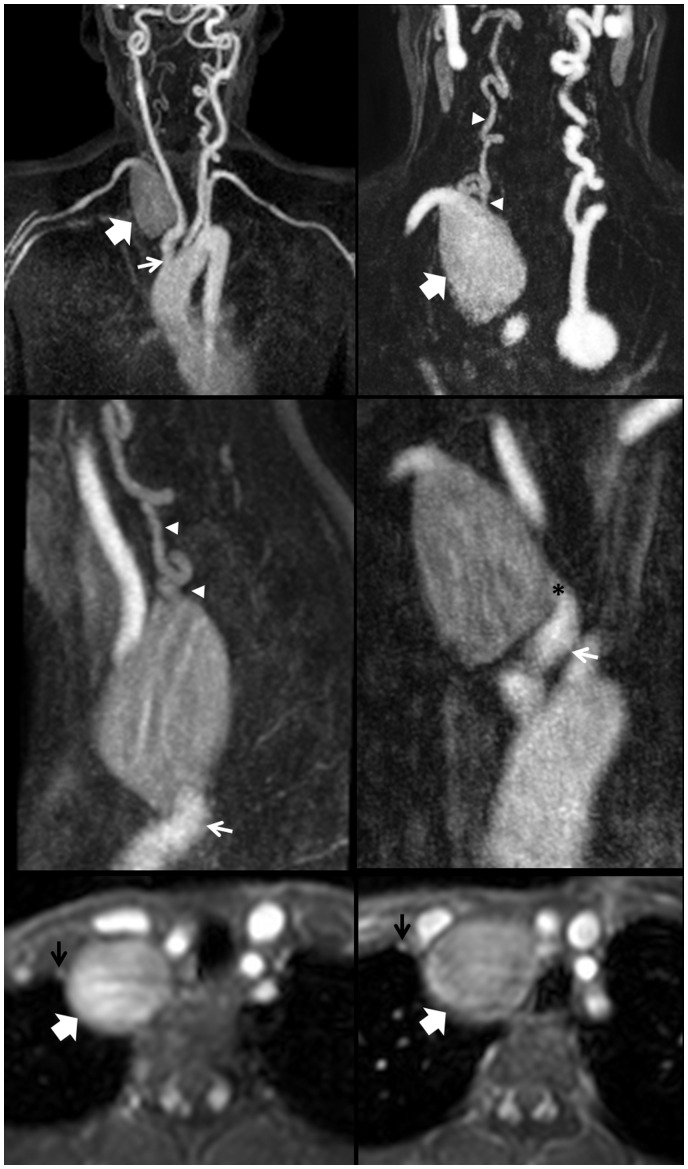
Contrast-enhanced magnetic resonance imaging with maximum intensity projection and multiplanar reconstruction showing large right subclavian artery aneurysm (large white arrows) and its entrance (black asterisk). The origin of the right vertebral artery from the aneurysm (white arrowheads) as well as the relationship to the brachiocephalic artery (small white arrows) and the right internal mammary artery (black arrows) is also demonstrated.
